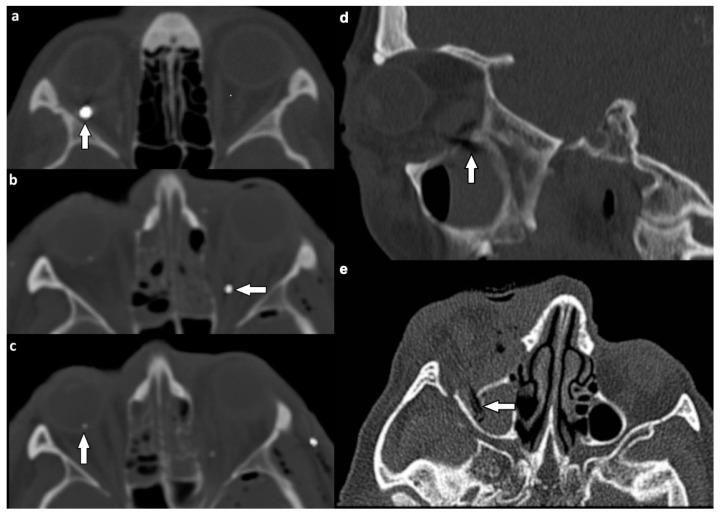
Intraorbital foreign bodies. NE-MSCT, bone window, axial reformation. (a) Metallic foreign body (white arrow) within the right lateral rectus muscle. (b,c) Multiple metallic foreign bodies in one patient (b) near the left optic nerve (white arrow) and (c) within the rear wall of the ocular globe (white arrow). (d,e) Wooden foreign body in the right orbit (white arrows) piercing through the orbital floor into the right maxillary sinus. Concomitant hematosinus and pronounced proptosis due to retrobulbar hematoma and swelling. Hypodense air bubbles entrapped within the otherwise undetectable dry wood. NE-MSCT, bone window and bone kernel in (d) sagittal and, (e) axial reformation (NE-MSCT = non-contrast-enhanced multi-slice CT).
